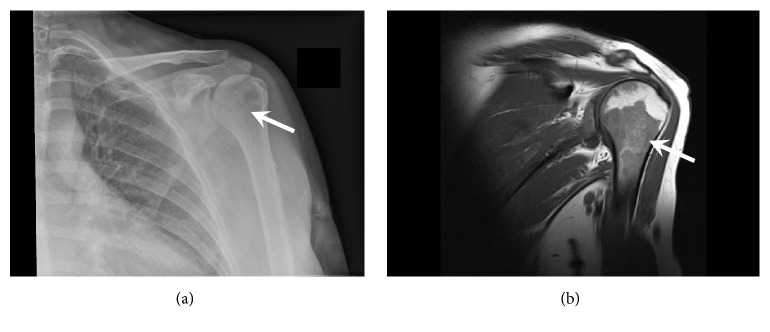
Imaging of left shoulder: (a) Plain radiograph showing lucency of humeral head. (b) MRI scan showing marrow infiltration of humeral head and shaft.
